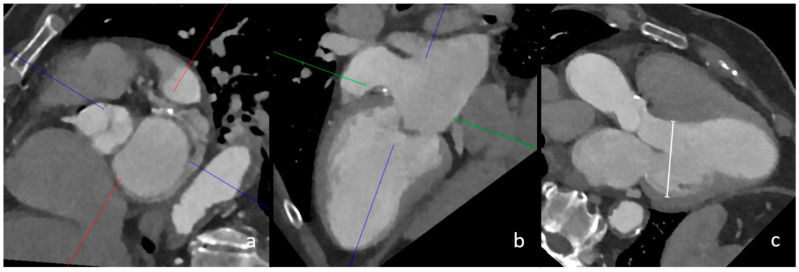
CT measurement of left ventricular dilatation in three-chamber view. In this image, the multiplanar reconstruction angulation of cCT imaging (a,b) is depicted. The diameter of the left ventricle in this female patient was 56 mm, which confirmed left ventricular dilatation (image (c), white line).
